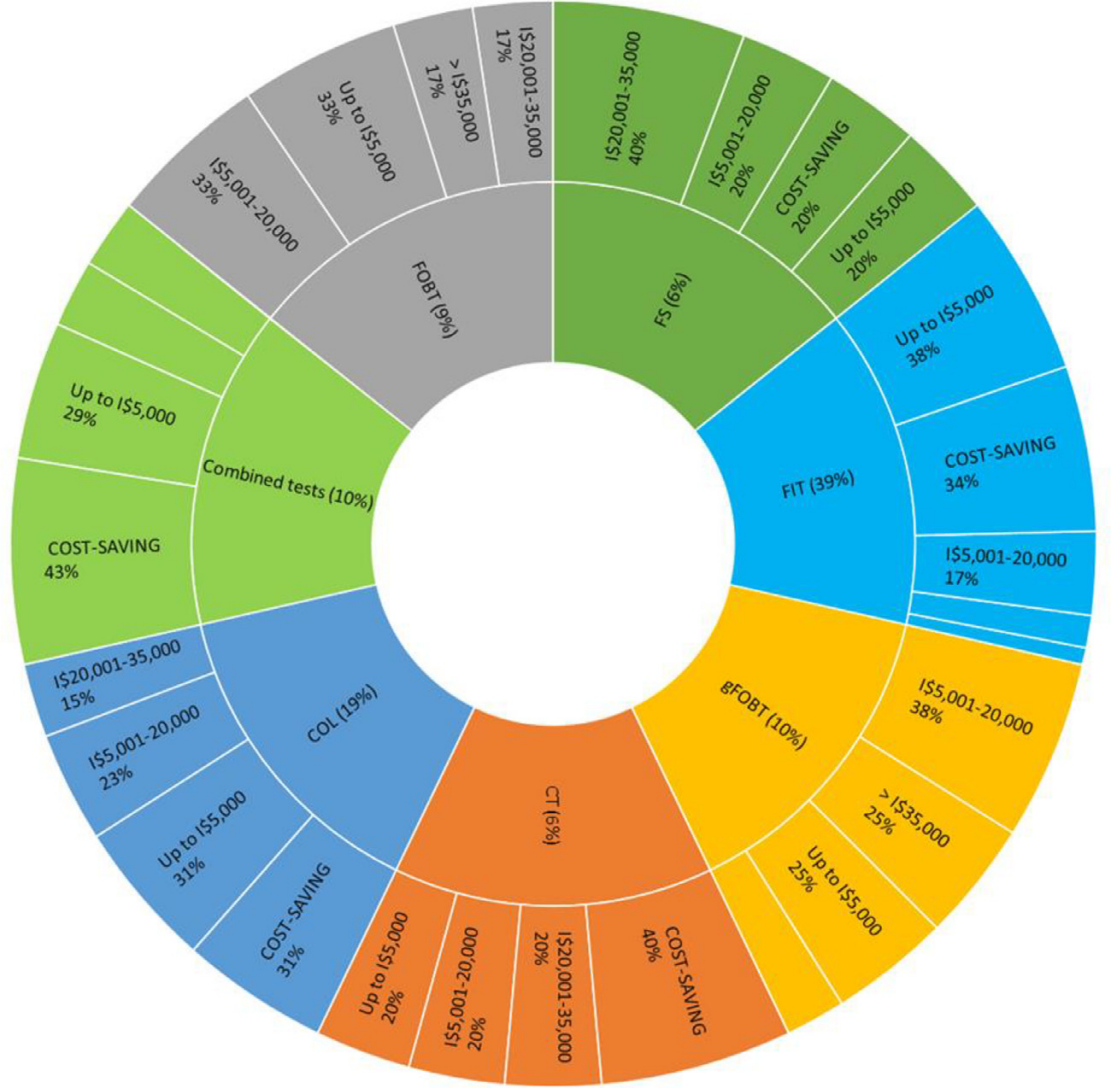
Colorectal cancer screening Incremental Cost-Effectiveness Ratios (ICERs) results. COL, Colonoscopy; CT, Computed Tomography; FIT, Fecal Immunochemical Test; FOBT, Fecal Occult Blood Test; FS, Flexible Sigmoidoscopy; Combined tests (When more than one type of test was used). *All ICERs were adjusted by local inflation to 2020 and then converted in International dollars (I$) using the Organization for Economic Co-operation and Development (OECD) Purchasing Power Parity conversion rates.
